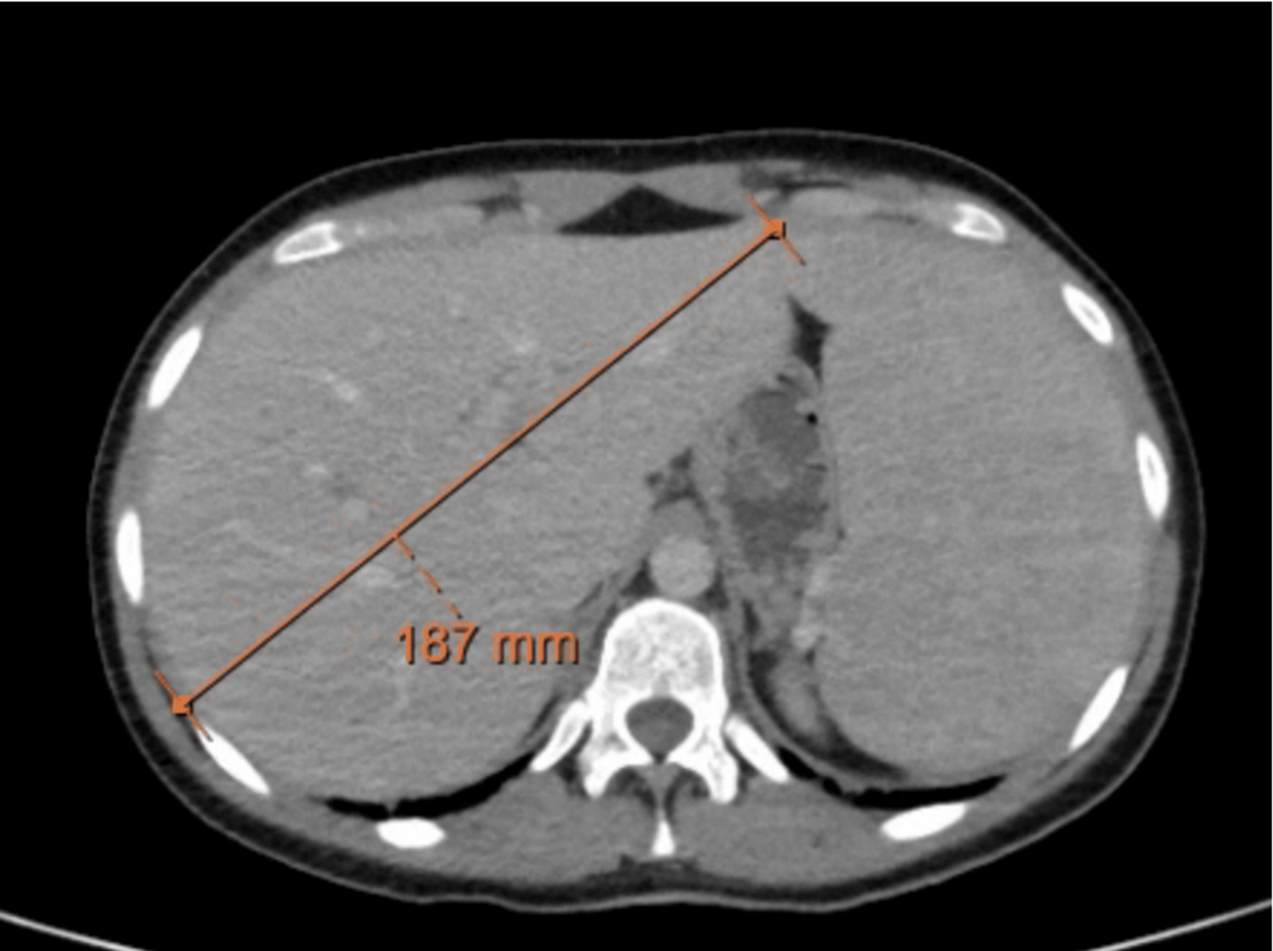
Axial plane of abdomen and pelvis computed tomography Heterogeneous hepatomegaly (18.7 cm)
